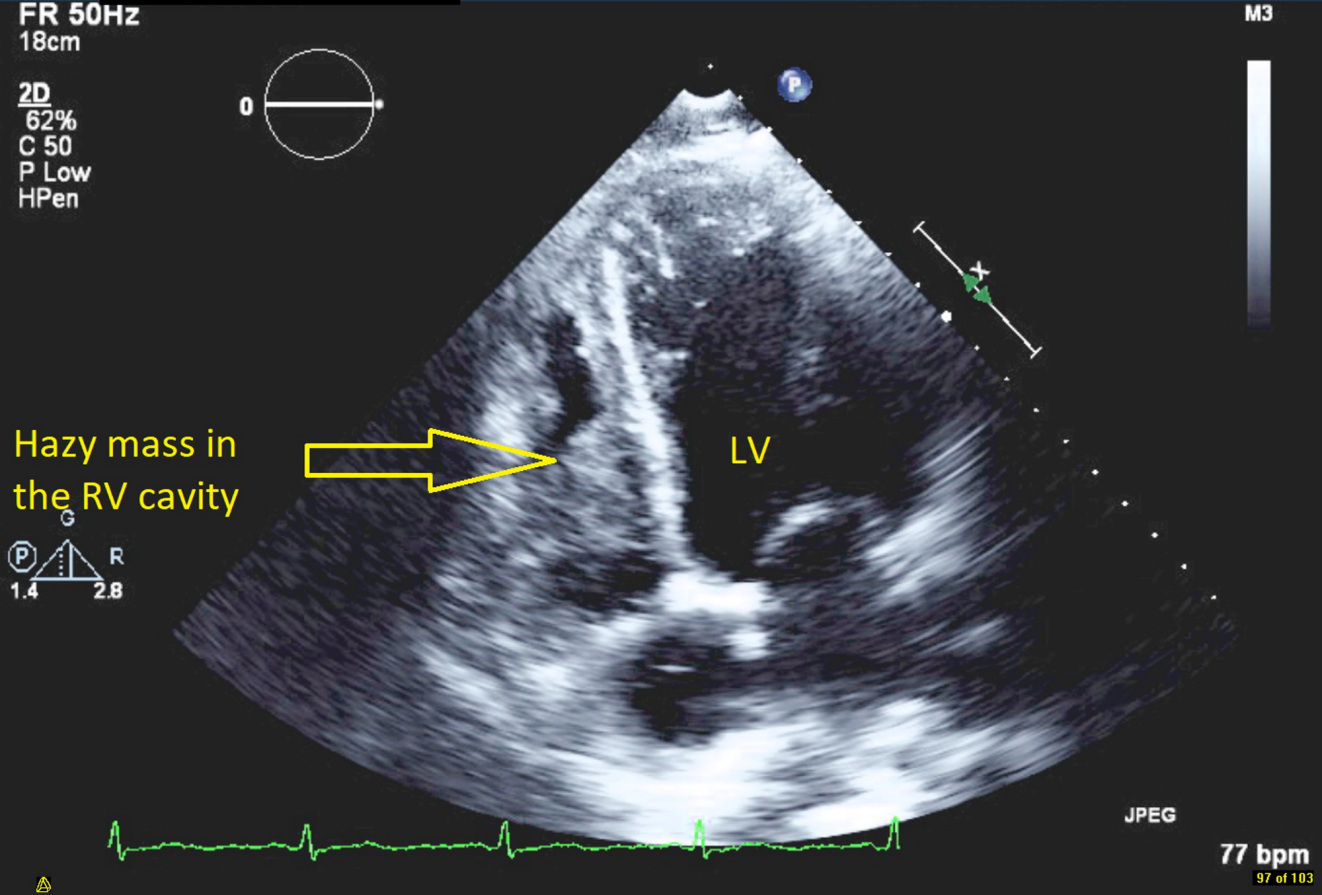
Transthoracic echocardiogram apical four-chamber view showing hazy density in the cavity of the right ventricle (RV) suggestive of a mass along with left ventricle (LV).
